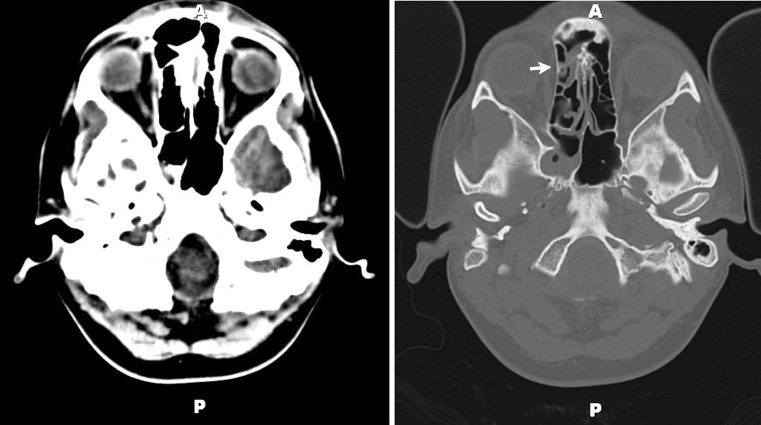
Non-contrast CT head. a An axial image showing mucosal thickening within the right sphenoid and ethmoid sinuses. Bone window from the same study more clearly demonstrating sinus disease (*white arrows*)
